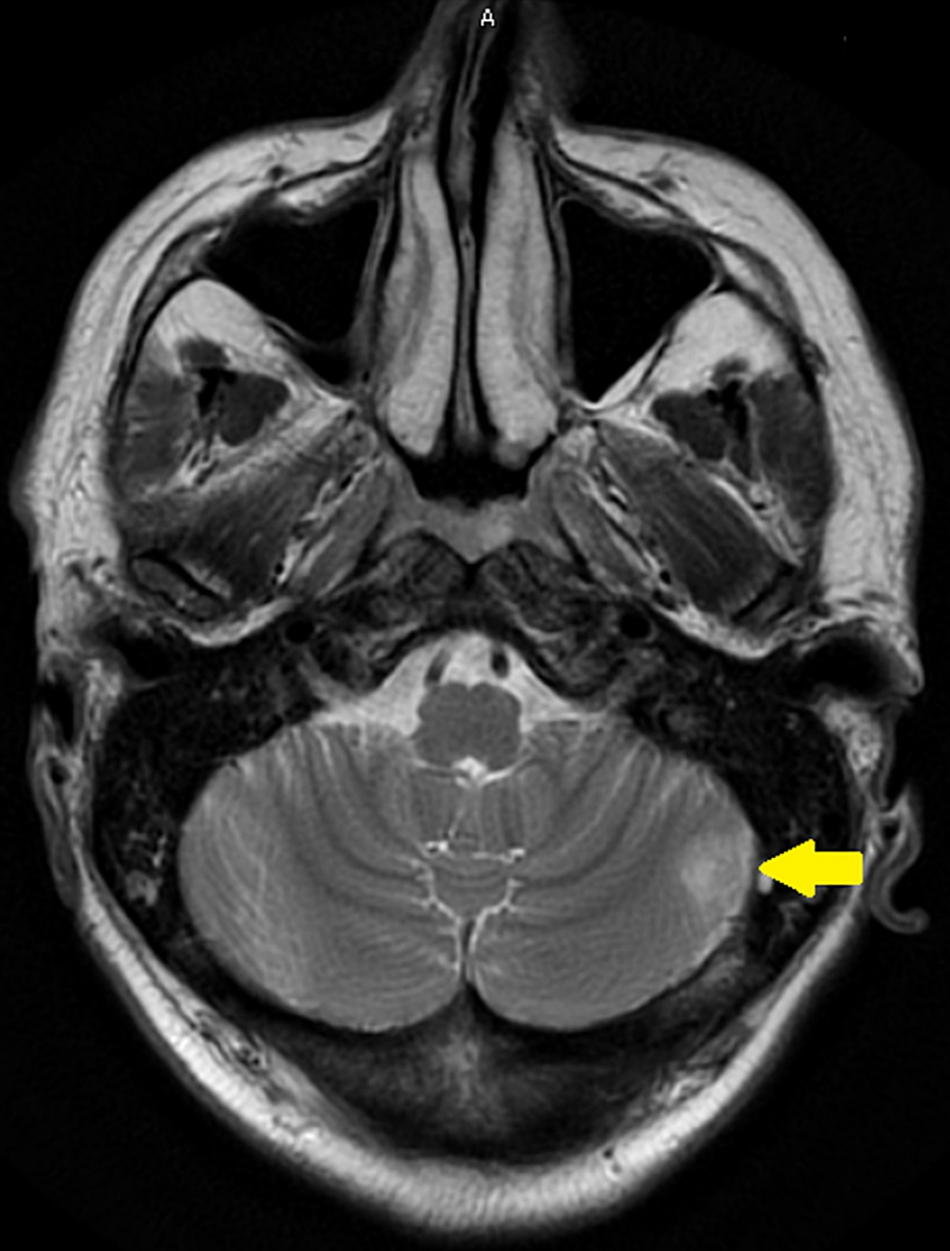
MRI brain with contrast showing a non-enhancing mass-like lesion in the left cerebellum.
